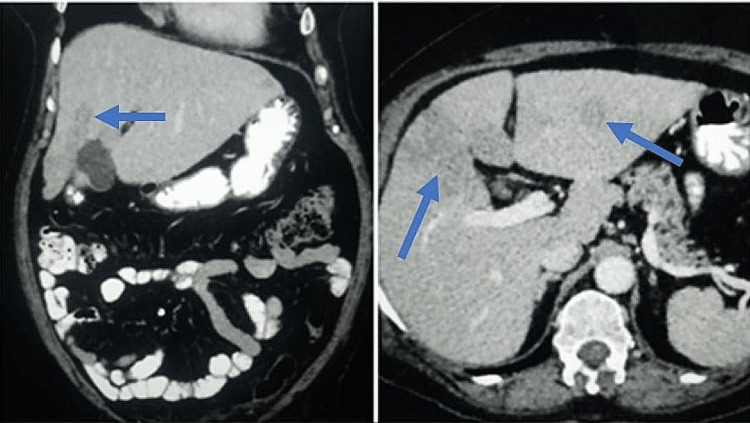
Abdominal computed tomography demonstrating hepatomegaly with mild hypoattenuation of liver parenchyma and multiple variable-sized peripherally enhancing hypodense lesions in both lobes of the liver.
